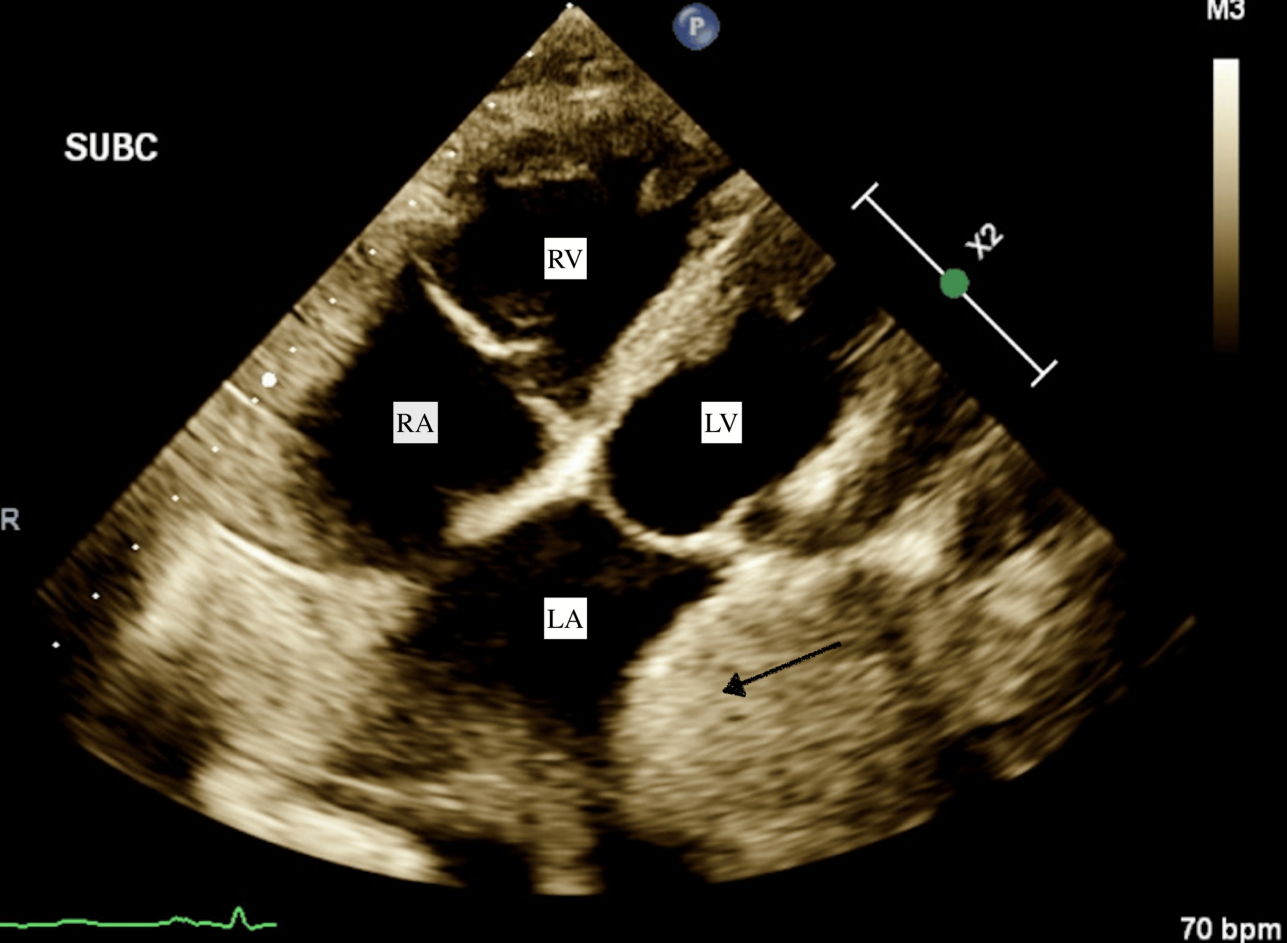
Echocardiographic subcostal 4 chamber view The arrow indicates an infiltrative lung mass compressing the left atrium (LA).
